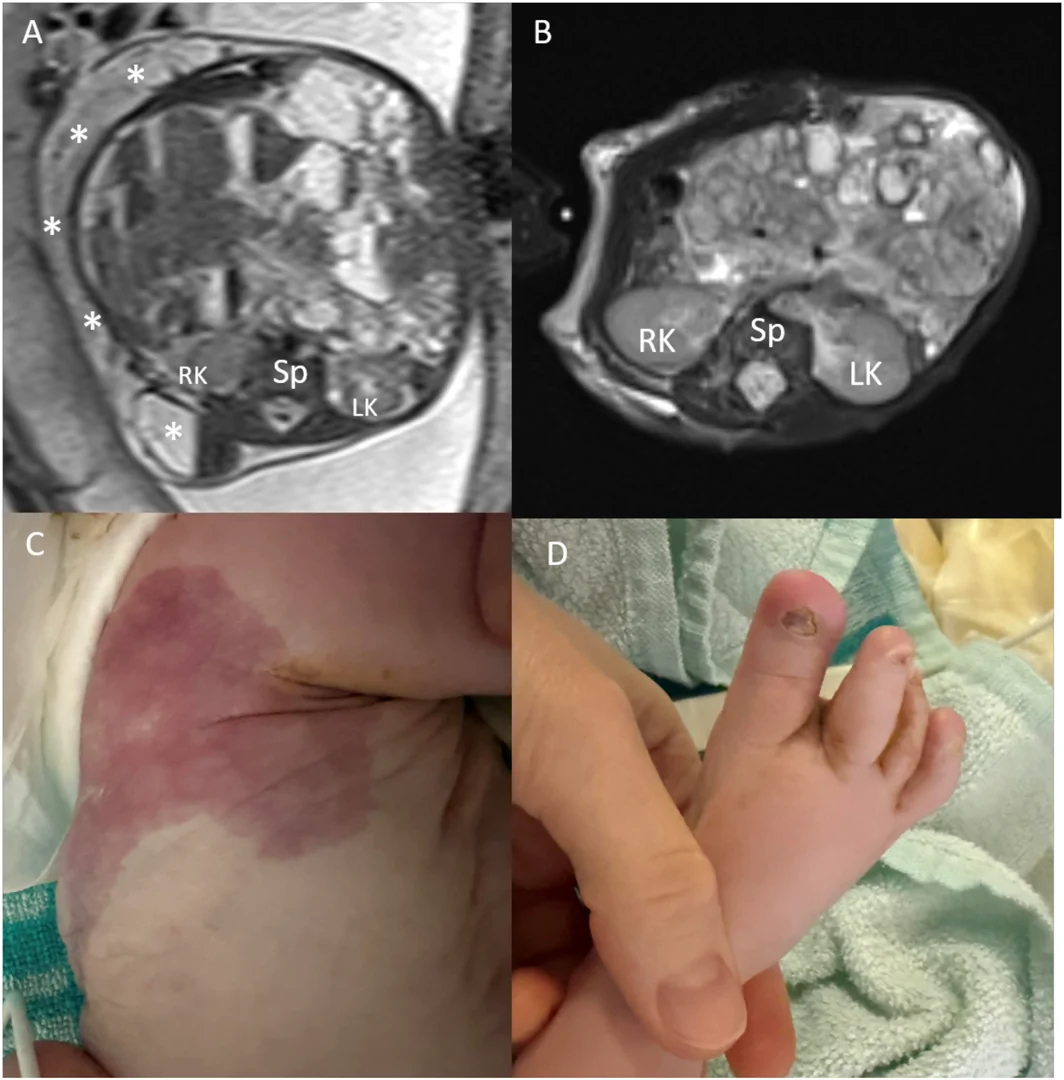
(A‐D): Case 5 with MST at 30 gestational weeks with a good outcome (graded 3). Fetal axial T2 weighted MR image at 28 gestational age at the level of the right and left kidneys (RK, LK) showed an extensive involvement of the whole peritoneal cavity by numerous cystic lesions with fluid‐fluid levels suggestive of internal cystic hemorrhage with diffuse involvement of the right abdominal wall (white *) without clear identification of the intestinal loops (Sp: spine). Post‐natal imaging at the same anatomical level showed a major decrease in both intraperitoneal cysts and abdominal wall infiltration. Neonatal clinical examination showed a large capillary malformation facing the right hemithorax which could not be diagnosed prenatally, which anomalies of the extremities of the left foot, including macrodactyly involving the first and second toes, as well as a sandal gap (excessive distance between the first and second toes), which were overlooked on prenatal imaging.
